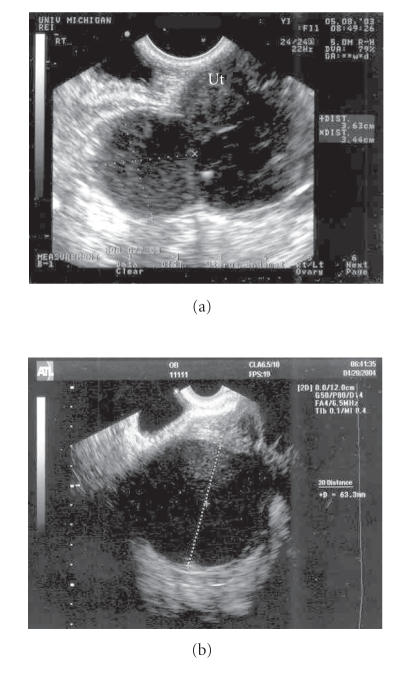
(a) Transvaginal ultrasound image, shortly
